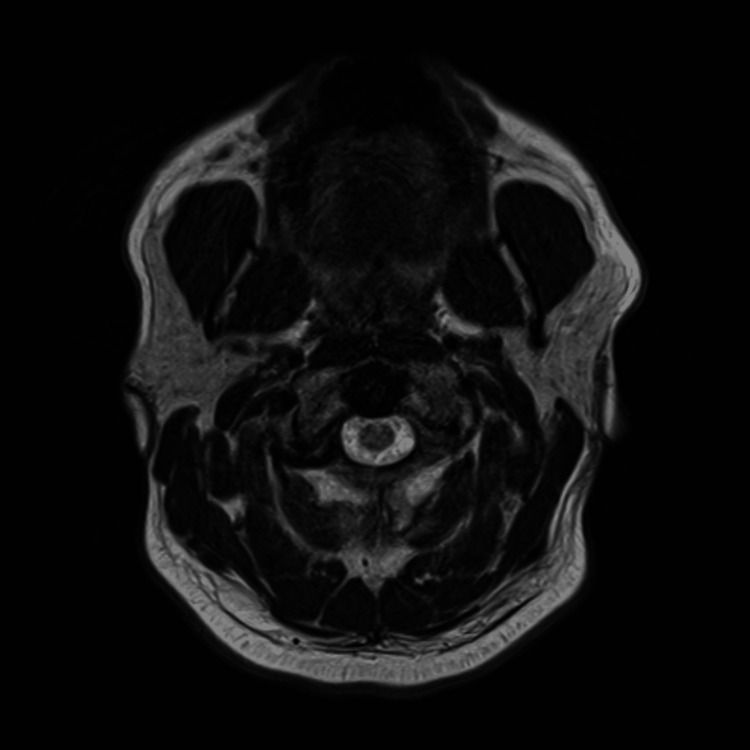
Normal magnetic resonance imaging of the brain with and without contrast No acute intracranial abnormality detected on this motion-degraded examination.
